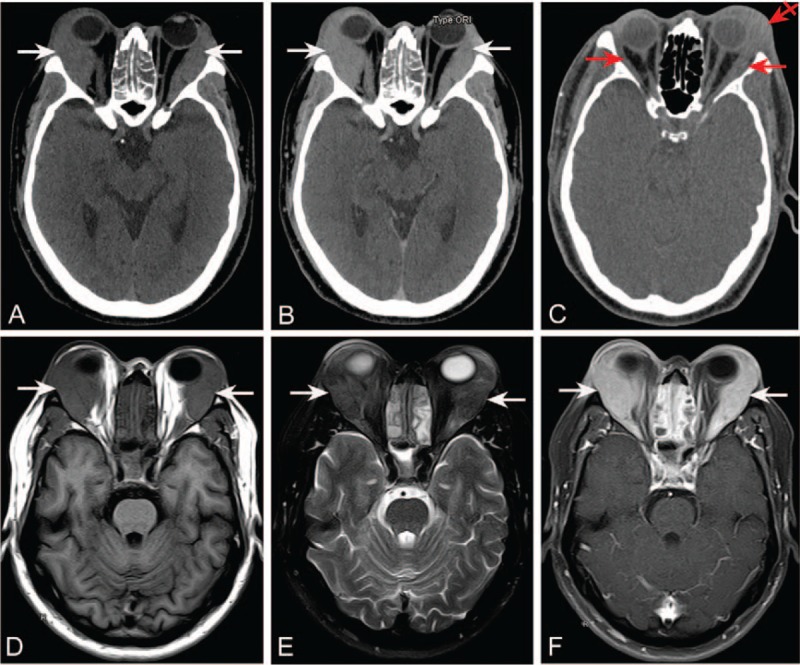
Cranial axial CT (A: non-enhanced image; B: enhanced image) and MRI (D: T1WI-FLAIR; E: T2WI; F: contrast-enhanced T1WI) demonstrate significant homogeneously enhancing soft-tissue masses (white arrows) in the bilateral orbits and crowding of the optic nerves, especially on the right side. Cranial axial CT four months later (C: non-enhanced image) reveals remarkable narrowing of the bilateral soft-tissue masses (red arrows) and slight enlargement of the left lacrimal gland (red crossed arrow). CT = computed tomography; MRI = magnetic resonance imaging; T1WI = T1-weighted imaging; FLAIR = fluid-attenuated inversion recovery; T2WI = T2-weighted imaging.
